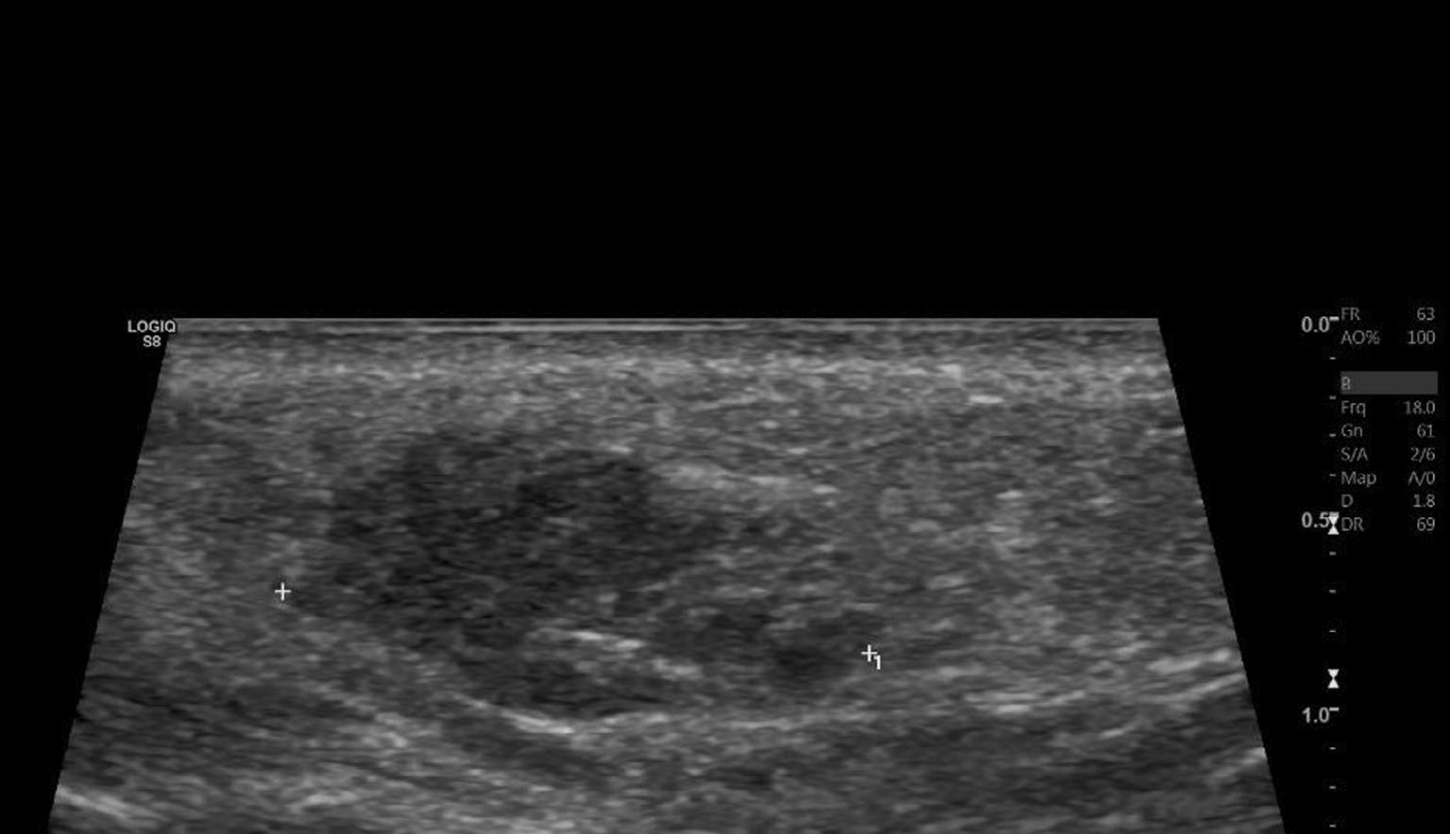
Ultrasonographic findings in case 1 demonstrating bilateral lobulated hypoechoic nodules within the subcutaneous tissues of the nasolabial folds, most likely representing a granulomatous reaction to soft tissue fillers.
